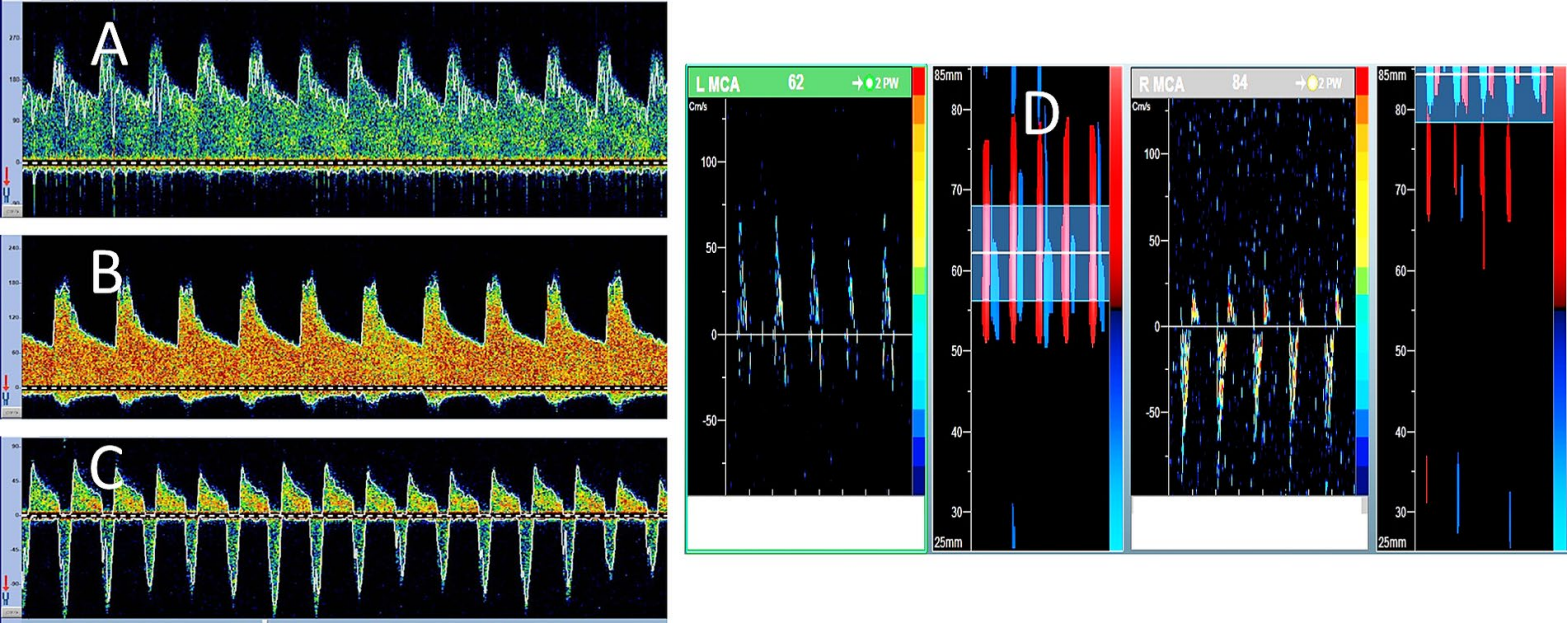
Serial transcranial Doppler (TCD) findings in brain death. A 48-year-old woman was admitted with severe subarachnoid hemorrhage due to an anterior communicating artery aneurysm rupture. She underwent urgent clipping and was monitored regularly with TCD. Her level of consciousness remained poor, while ICP continued to increase despite all therapeutic measures. During the 7 days of follow-up, his TCD spectra showed serial evolution from a normal-looking waveform (A) on day 2 to a higher resistance waveform (B) on day 4 to the oscillating pattern (suggestive of cerebral circulatory arrest) on day 5 (C). No brainstem reflexes were observed, and TCD spectra showed further evolution of the oscillating Doppler spectra in both middle cerebral arteries (D).
